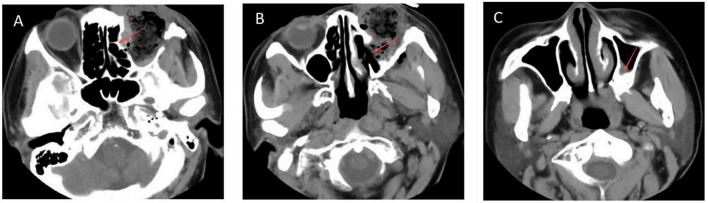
Postoperative orbital CT scan with three-dimensional reconstruction revealed localized inward depression of the medial wall of the left ethmoid sinus (A) and the superior wall of the left maxillary sinus (B), accompanied by minimal exudative changes in the left maxillary sinus (C).
